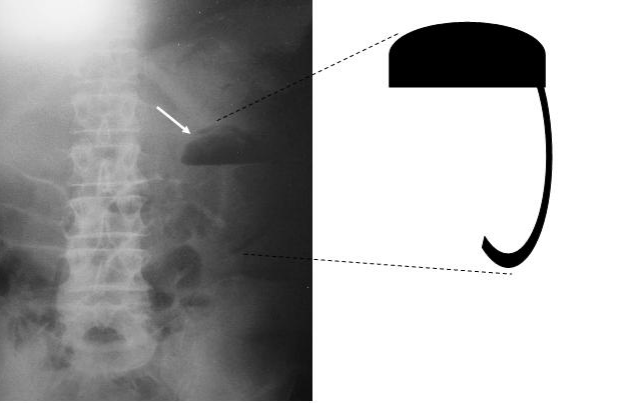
Abdominal radiograph of the 'comma sign' in our patient.
